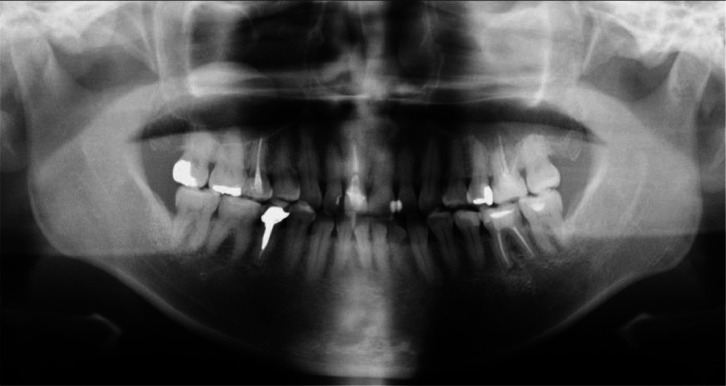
Panoramic radiograph. No sialolithiasis is seen and there are no notable findings.
